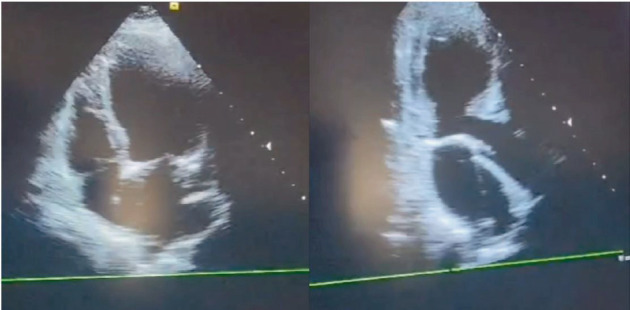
The echocardiogram taken just after the onset of chest pain shows mild left ventricular enlargement, reduced ejection fraction, and akinesia in the anteroseptal and apicoseptal walls.
